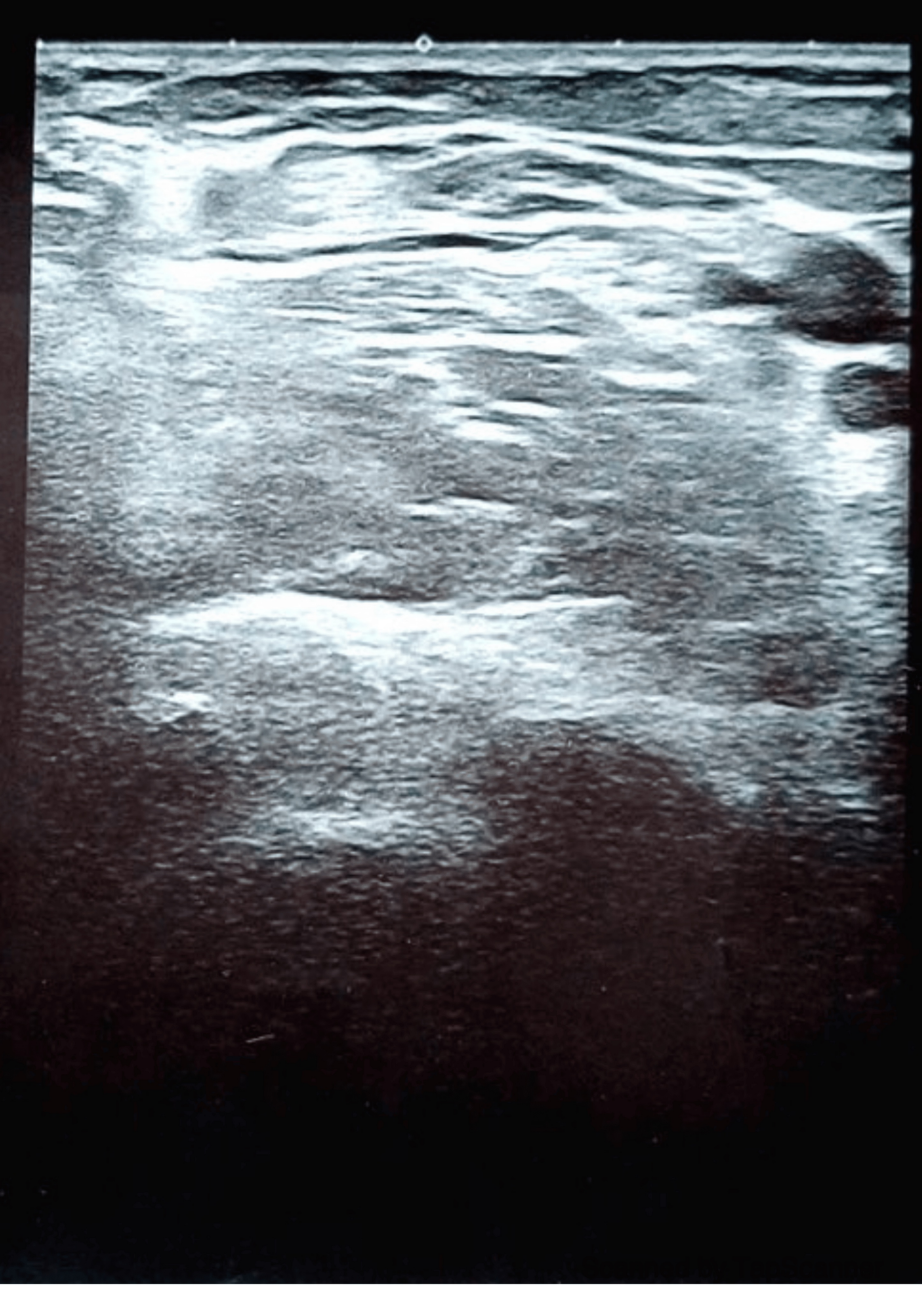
Ultrasound image of the tumor bed 14 years after the excision
